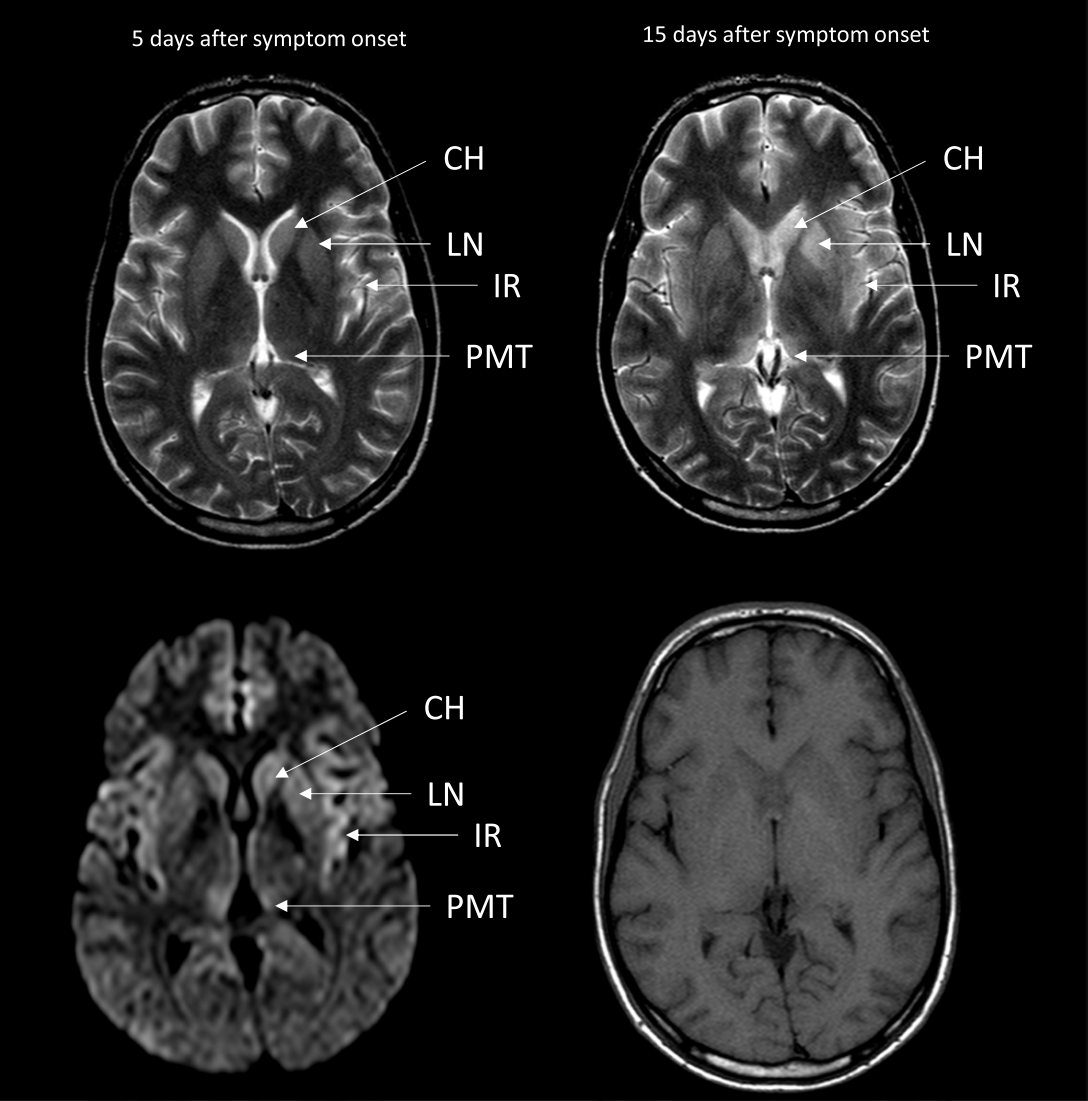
Typical cranial magnetic resonance imaging of early BoDV-1 encephalitis. Left column: T2 weighted imaging (top left) shows discrete, nearly symmetric hyperintensities of the insular ribbon (IR), the posteromedial thalamus (PMT), the caudate head (CH) and the lentiform nucleus (LN). These changes are more easily appreciated on diffusion-weighted imaging (DWI, bottom left), where diffusion restriction in these brain areas is visualized as a hyperintense signal. Right column: T2 weighted imaging (top right) shows progressive T2 hyperintensities of the affected areas with associated parenchymal swelling, somewhat more pronounced on the patient’s left side. No T1 hyperintense signal changes, which are characteristic of late-stage BoDV-1 encephalitis, were present at this time point (bottom right)
